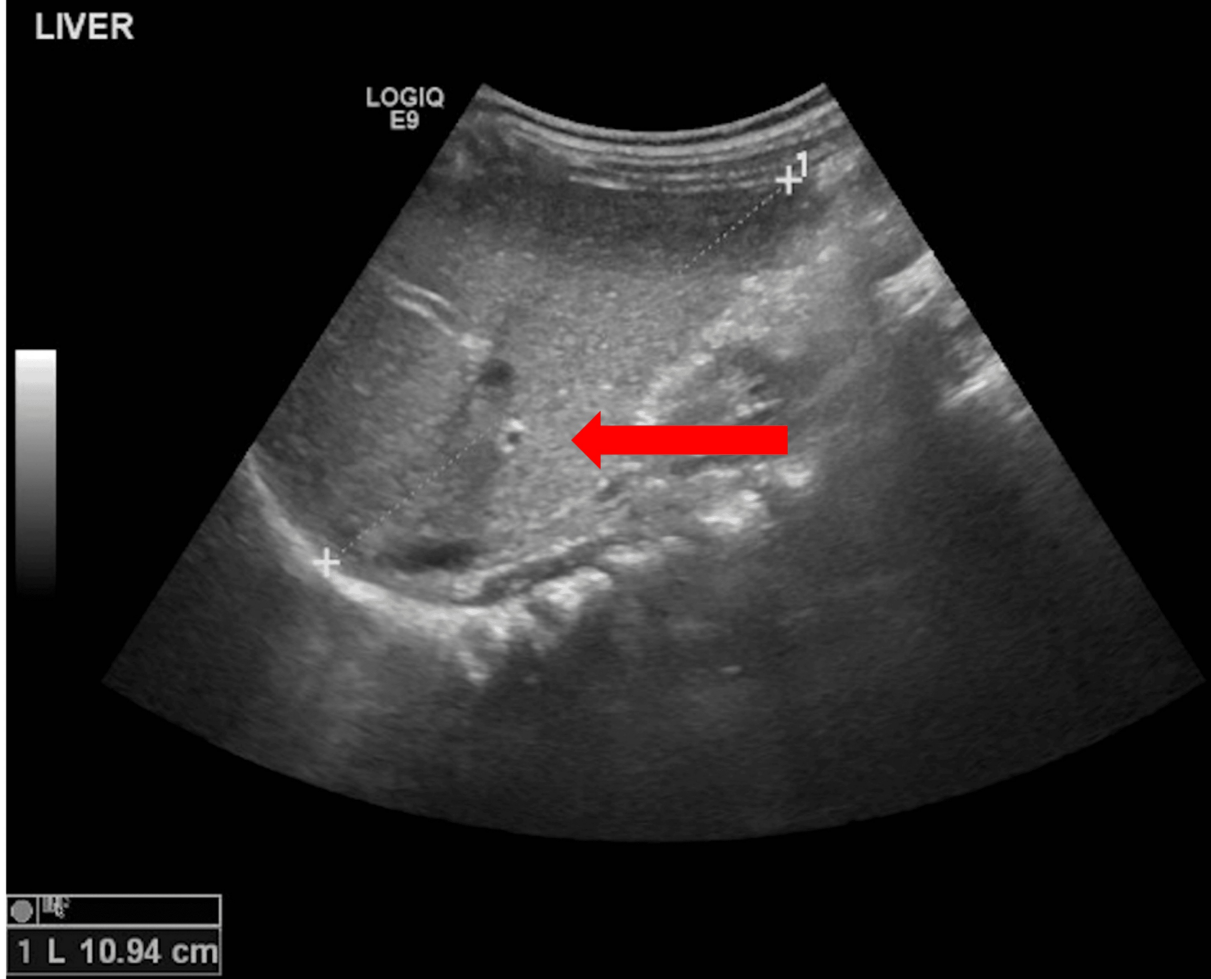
Ultrasound of the abdomen Ultrasound image showing hepatomegaly with the liver measuring around 11 cm along its long axis (arrow).
